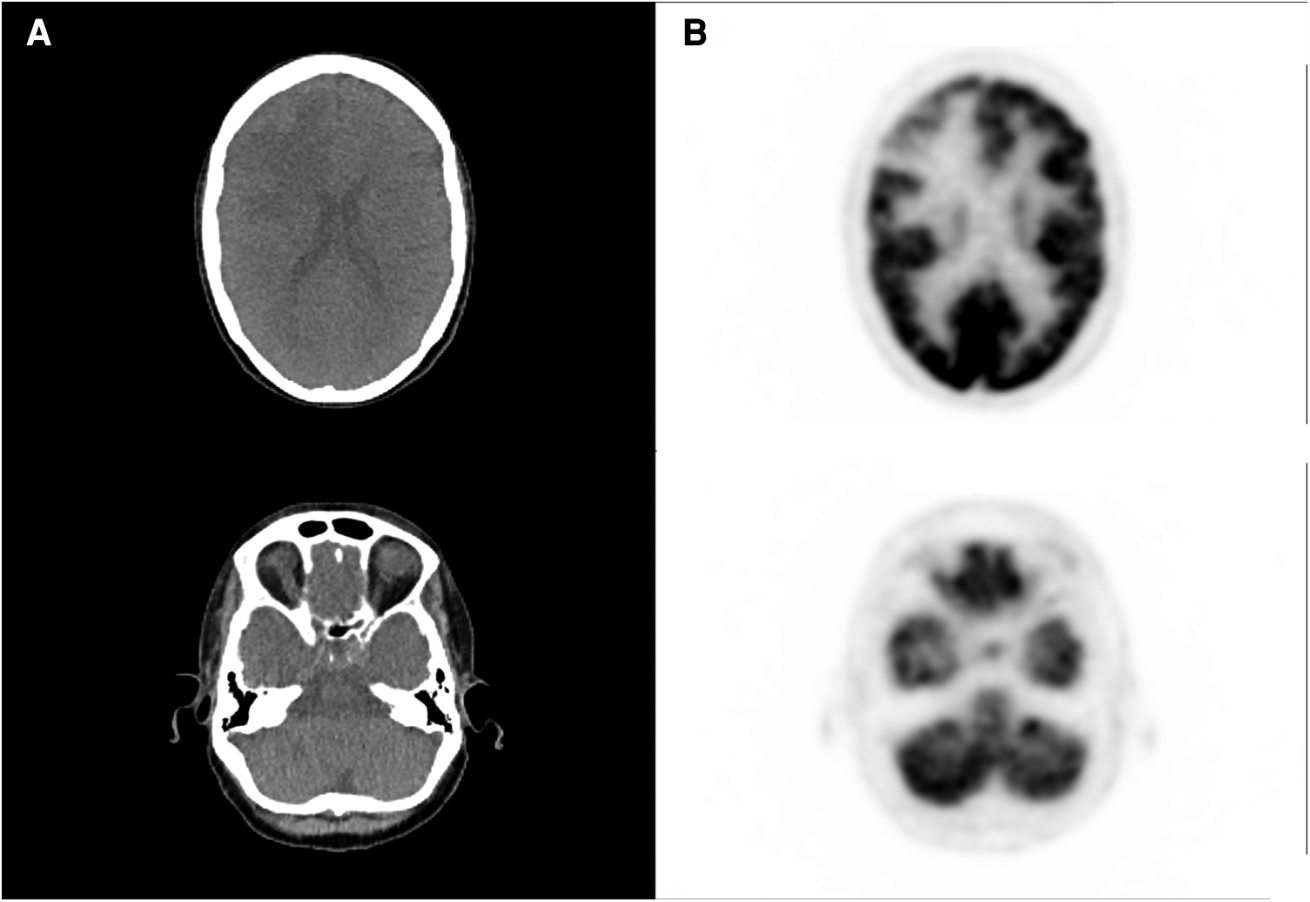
[^18^F]FDG PET and CT acquisition performed after the first doubtful MRI, showing large hypometabolic right frontal area (A) and no significant abnormal uptake in the left cerebellar region (B).
